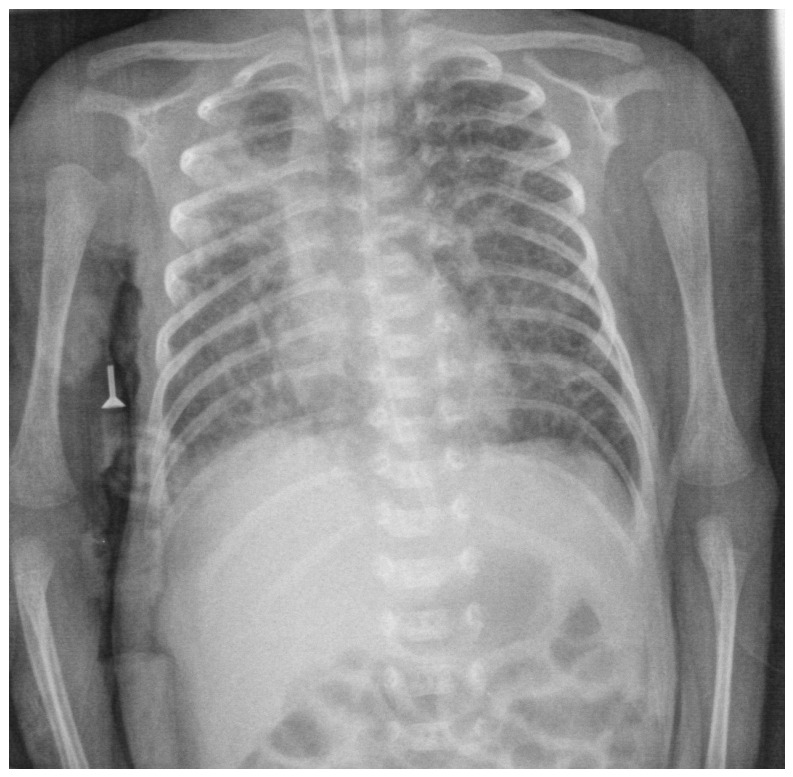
Chest X-ray showing bilateral, irregular loss of transparency of the lung parenchyma.
